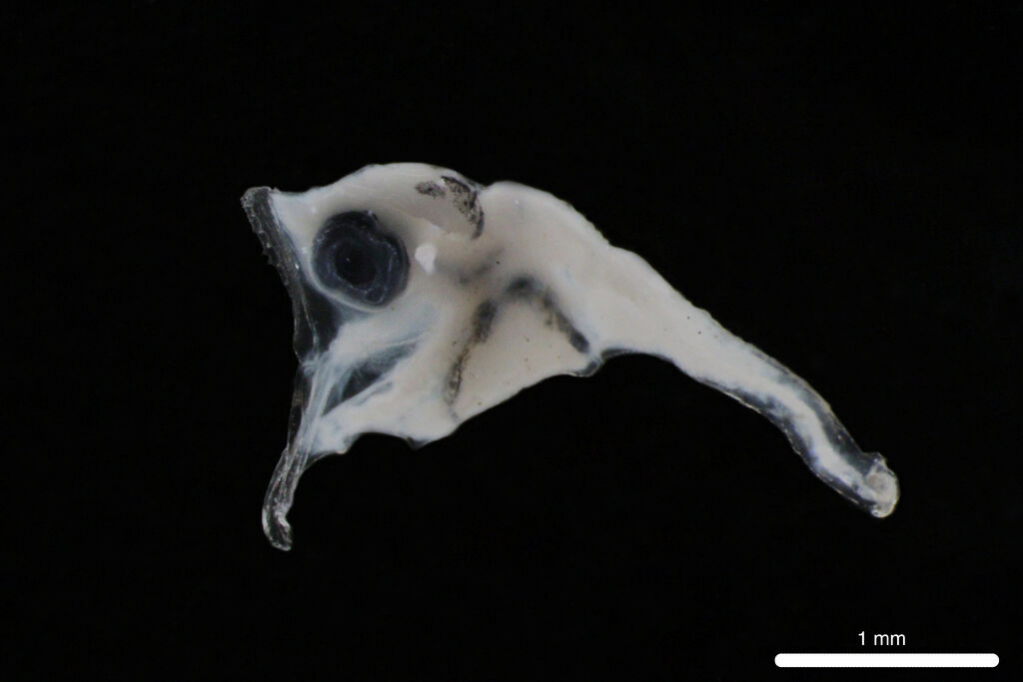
DL323 captured with PN in the CTA during PN_007.
